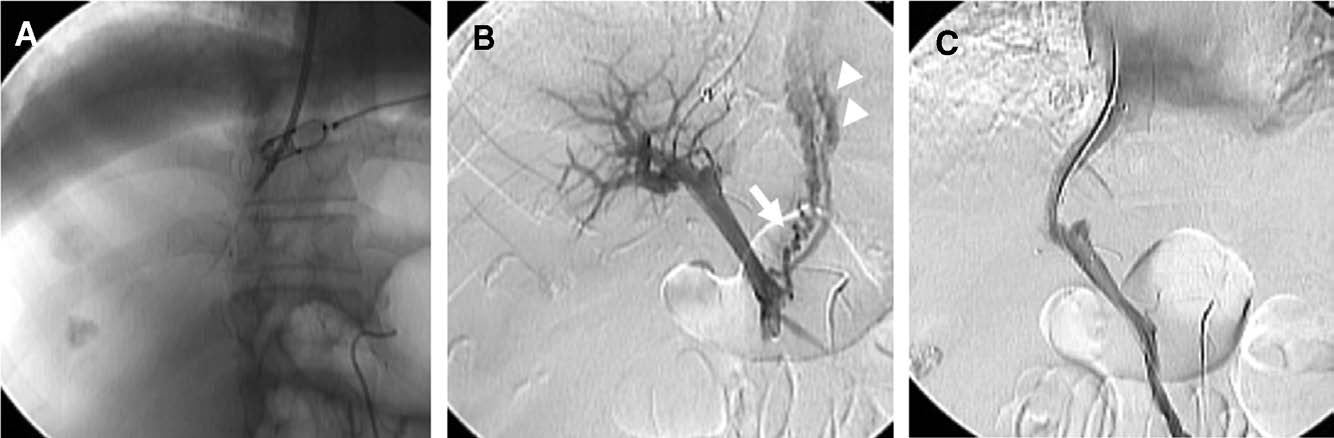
(A) The 3D roadmap is fused with the working fluoroscopy screen. (B) Digital substraction portal venogram prior to TIPS procedure depicts a left gastric vein (arrow) supplying esophageal varices (arrowhead). (C) Abdominal radiograph after the TIPS procedure. Esophageal varices are no longer visualized. TIPS = transjugular intrahepatic portosystemic shunt.
